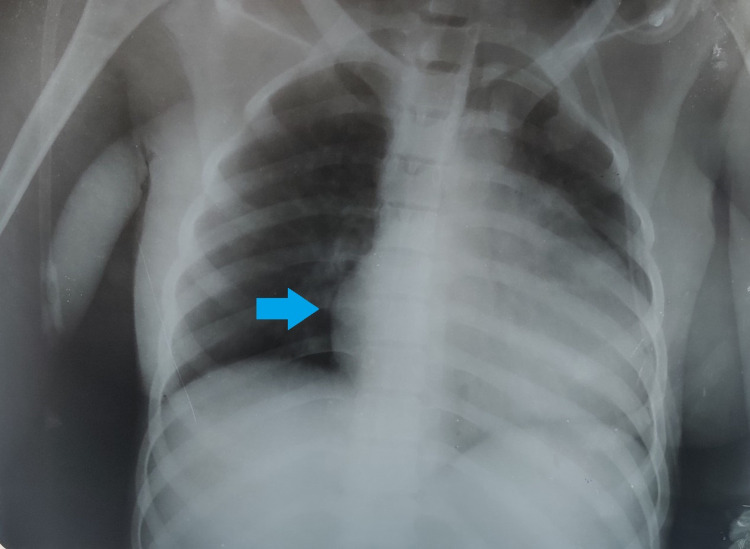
Plain chest X-ray of the patient showing bilaterally enlarged cardiac silhouette (arrow)
